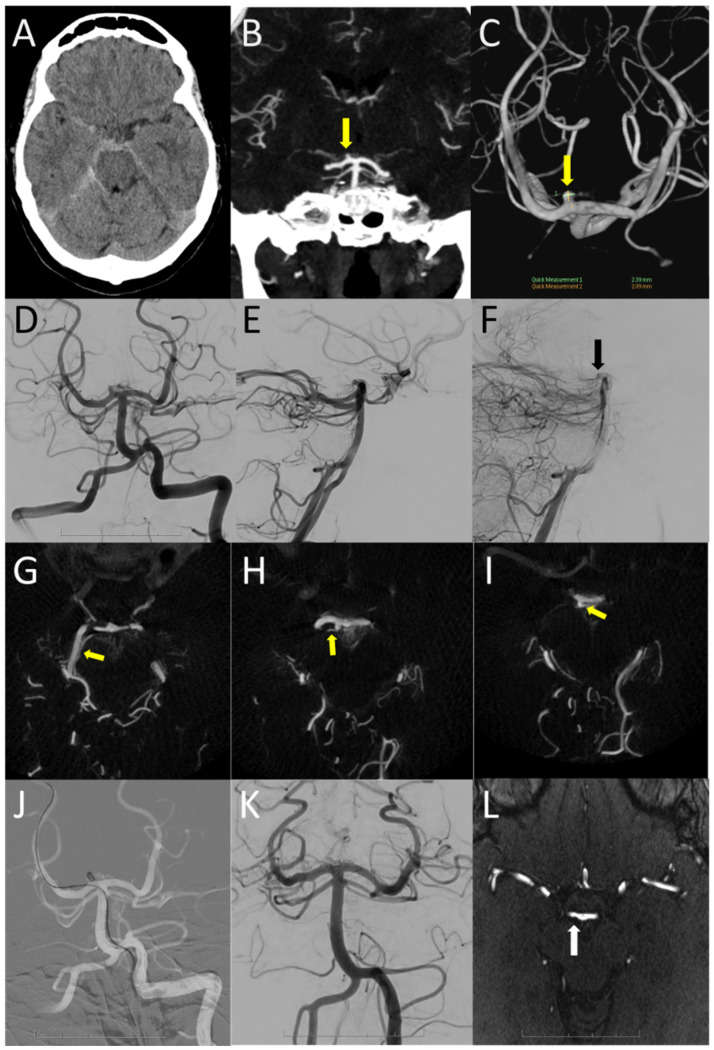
Case no 13 involves a 64-year-old female patient with a subarachnoid hemorrhage (GCS 15, WFNS 1) with the epicenter in the right interpeduncular cistern (A), with suspicion of a small superiorly and posteriorly pointing right P1 aneurysm ((B,C); yellow arrow). AP (D) and lateral left vertebral artery injections (E,F) confirm a right P1 aneurysm, with stases of contrast in the dome in the late arterial phase ((F); black arrow). Axial Xpert CT angiography reconstructions demonstrate the course of the right collicular artery in the crural and ambient cisterns along the posterior cerebral artery ((G–I); yellow arrows) originating medially from the dome of the aneurysm ((I); yellow arrow). An unassisted coiling with balloon presence was performed with two coils (J) resulting in direct (K) and one-year follow-up closure of the aneurysm on MRA time-of-flight (L). There is subtle indentation of the coil mass in the parent artery ((L); white arrow).
